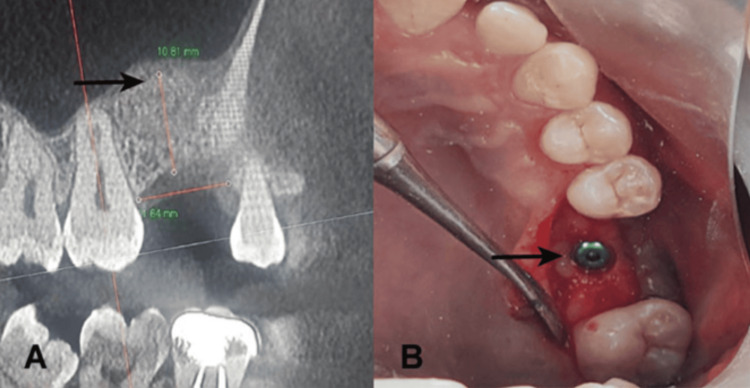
Post-operative CBCT showing an alveolar height of 10.8 mm in the area of #26 (A) and dental implant placement for #26 (B) CBCT: cone beam computed tomography
